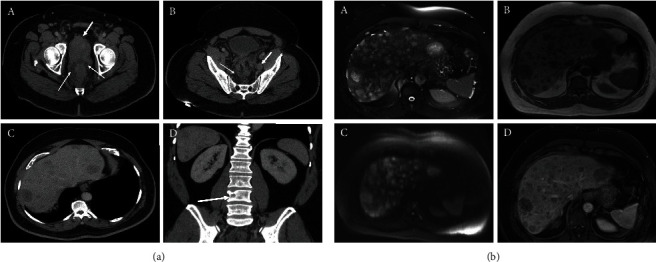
(a) CT images: postcontrast (A–C) axial and (D) coronal images showing the significantly enlarged heterogeneous prostate gland with irregular borders (thick arrow, A) and significant stranding involving the mesorectum (thin arrows, A) with associated rectal wall thickening. Presacral soft tissue involvement characterized as significant stranding is also noted (thin arrow, B) with associated left pelvic side wall enlarged lymph node (thick arrow, B). The findings are suggestive of infiltrative prostate cancer involving extraprostatic adjacent tissues with local extension with metastatic adenopathy. (C) Numerous hypoattenuating liver lesions likely represent bilobar hepatic metastatic disease. A lytic metastatic bone lesion with associated mild pathologic fracture is also noted at the right side of the L4 vertebral body (arrow, D). (b) MRI images: (A) transverse T2-weighted fat-suppressed single-shot echo train spin echo, (B) T1-weighted in-phase spoiled gradient echo, (C) diffusion-weighted, and (D) postgadolinium hepatic venous phase T1-weighted fat-suppressed three-dimensional gradient echo images show numerous bilobar metastatic liver lesions with high T2 signal, low T1 signal, high diffusion signal, and postcontrast rim type of enhancement with associated mild internal hypoenhancement compared to the background liver.
